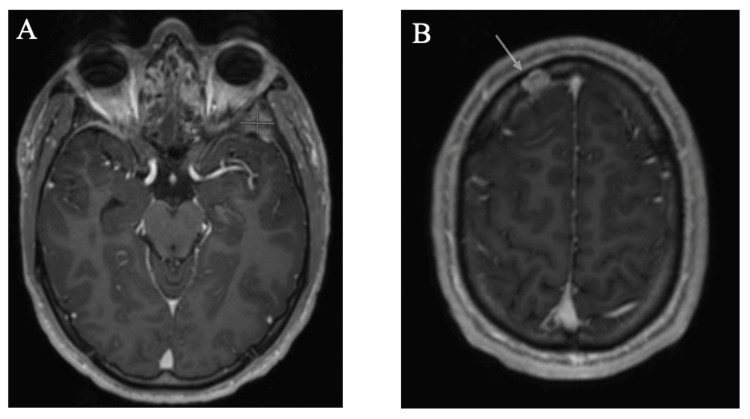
MRI brain findings after three cycles of cladribine. (A) Left sphenoid mass size reduction and resolution of mass effect. (B) New right frontal calvarium lesion (arrow) without intracranial extension. MRI, magnetic resonance imaging
